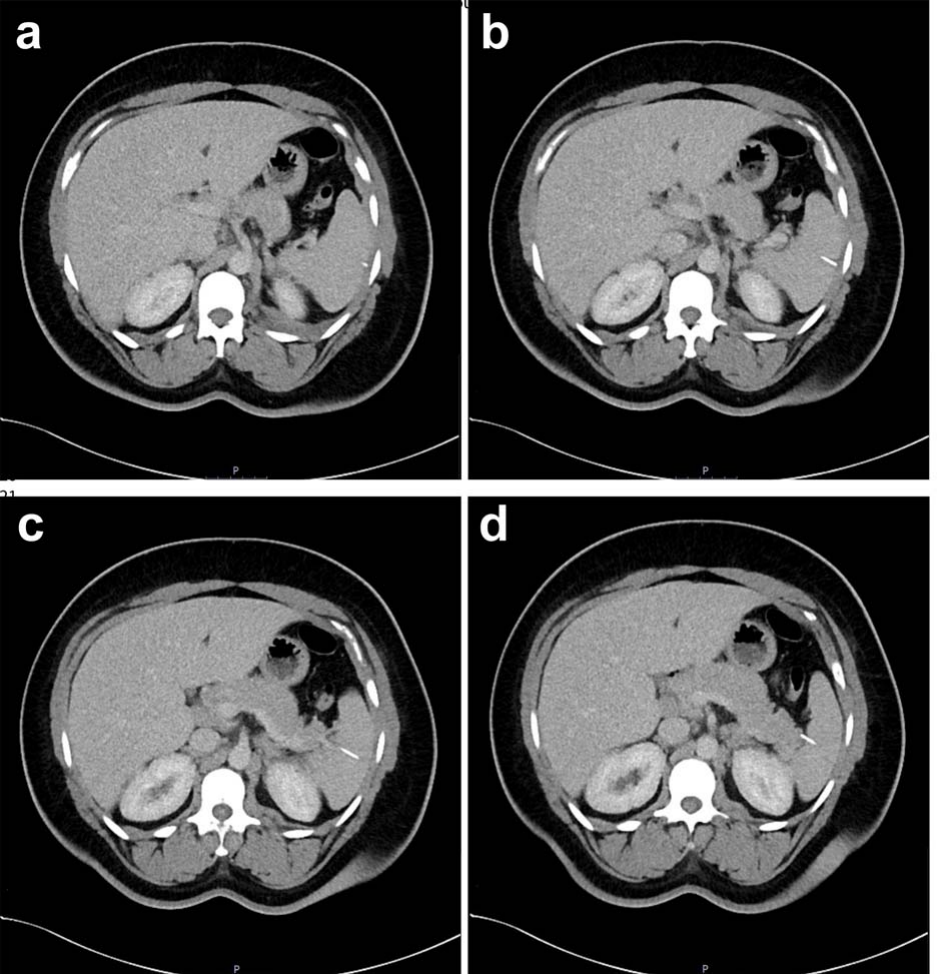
Two-mm axial sections from a CT scan in January 2020, performed after the VATS procedure, to check resolution of the empyema that shows the foreign body impacted in the spleen without evidence of haematoma. (a) section number 18, (b) section number 19, (c) section number 20, (d) section number 21.
